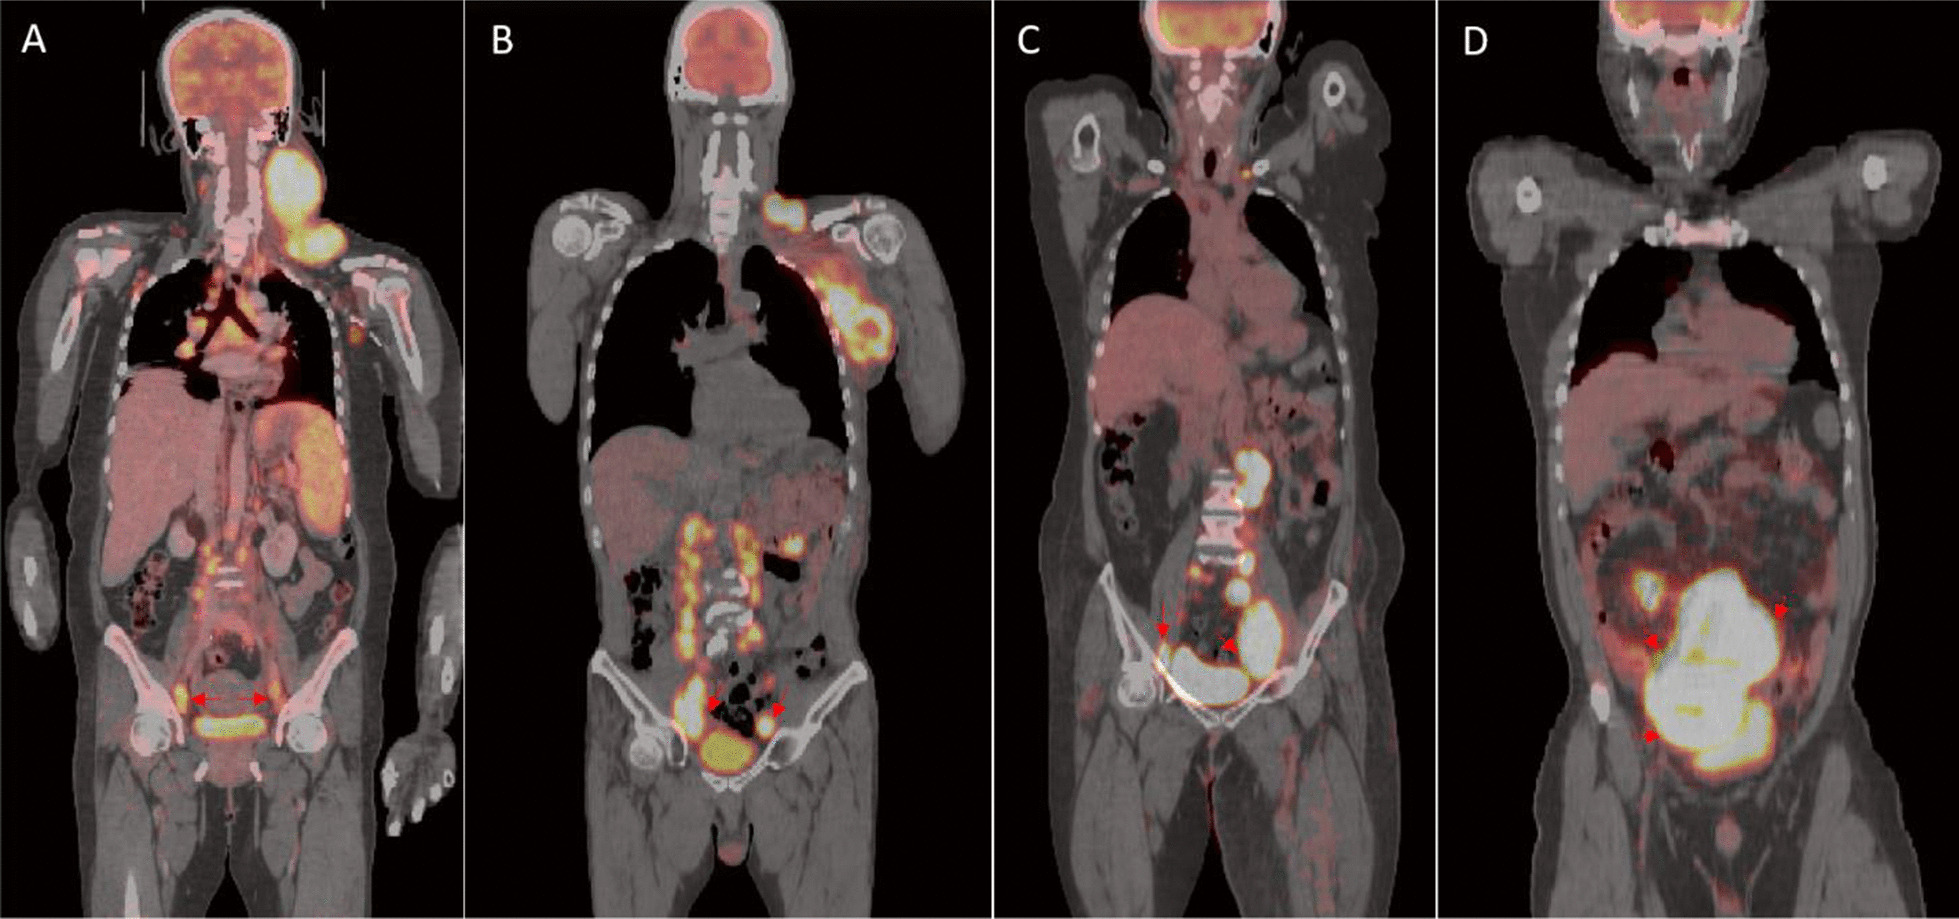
a, b Registered PET/CT showing cases where tumors are very close to the bladder. c, d Two cases with tumor connected to the bladder. Tumor is pointed out by red arrows
